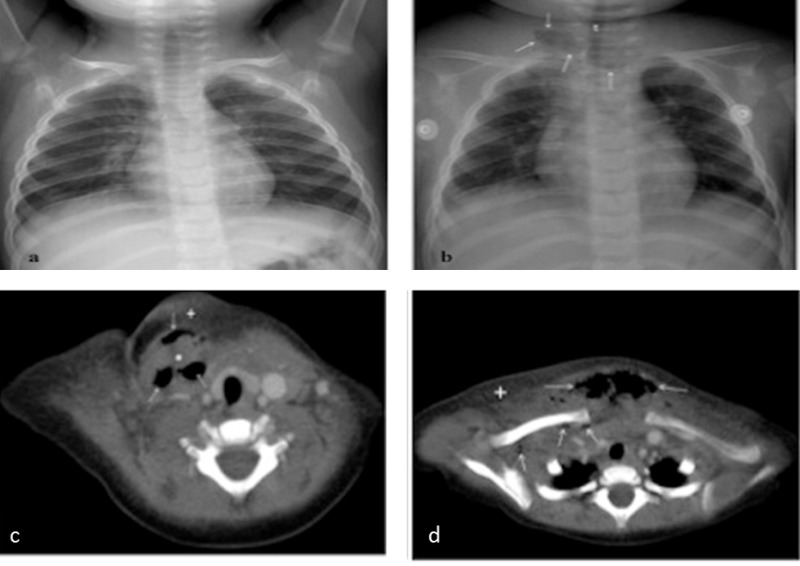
Figure 2: The chest radiography, that was normal on the referring day (a), shows air in the soft tissue (b) at the level of right cervical and inter-clavicular region on 5th day of admission (arrows). t: trachea. Contrast-enhanced axial CT shows an inflammatory swelling of the soft-tissue (*) with large abnormal air collections (arrows) suggesting abscess formation in the right cervical area (c), and spreads into the inter-clavicular region of the anterior chest wall (d). There is also subcutaneous edema (+)
